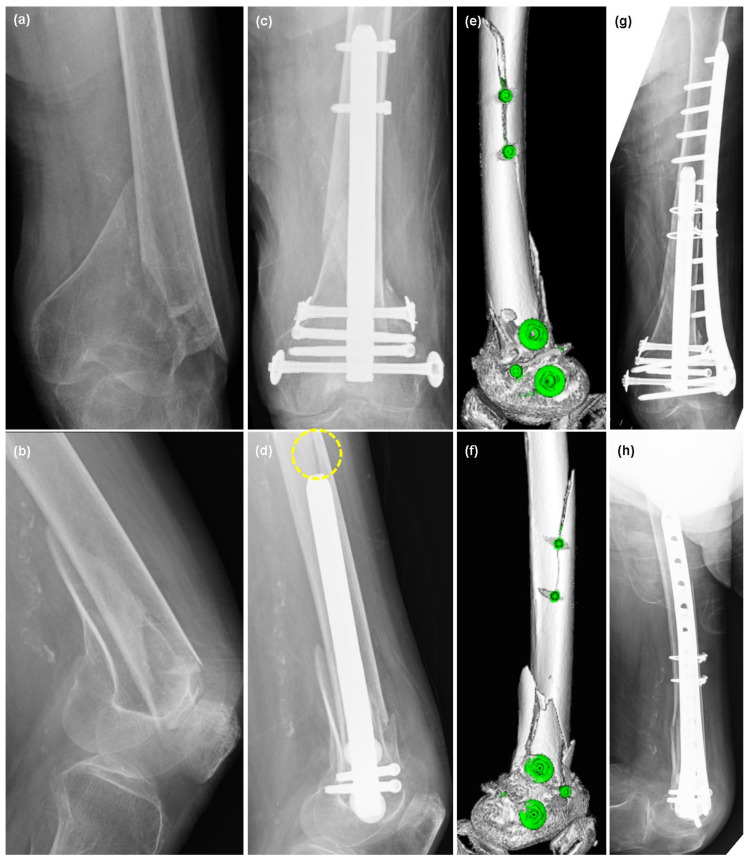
A 93-year-old female with a left supracondylar femoral fracture. (a) Preoperative frontal X-ray image of the distal femur. (b) Lateral image. (c) Postoperative X-ray frontal image. (d) Lateral image. The dotted circle shows the fracture line. (e,f) Postoperative CT images. The fracture was along the proximal advanced locking screws. (g) Re-fixation with distal femoral lateral plate (LCP DF, DePuy Synthes) and cable wires (DePuy Synthes). Front image. (h) Lateral image.
